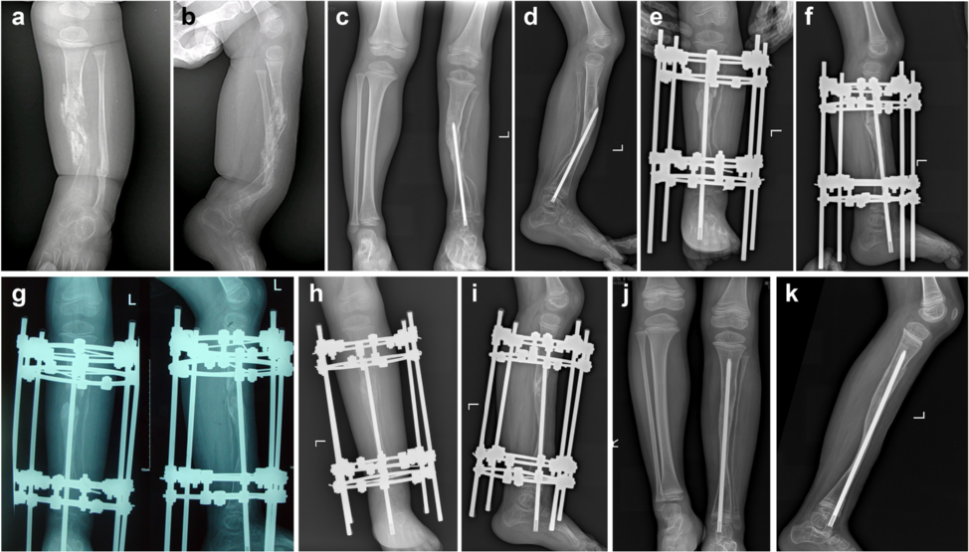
A 2.1y boy (Patient 4) with CPT of the left had two procedures of the tibia in another hospital previously. Radiographs showed the fragmental bone grafting but nonunion of the pseudarthrosis (a, b). Two years after combined surgery with Ilizarov fixator, intramedullary rodding of the tibia and wrapping autogenic iliac bone graft, the radiography showed a primary union of pseudarthrosis with LLD of 2 cm and angulation of tibia (c, d). A proximal tibial osteotomy and lengthening was carried out to correct the angulation deformity and equalize the limb length (e, f). Lateral callus was observed in this patient (g). Lengthening procedure was suspended for 2 weeks until new regenerate bone formation was observed around the callus. The length gained was 3.8 cm with HI of 63 d/cm (h, i). X rays of the last follow-up showed solid union of the lengthening segment and good alignment of tibia (j, k). The next step is to push the whole rod into tibia cavity when there is enough length in proximal tibia
